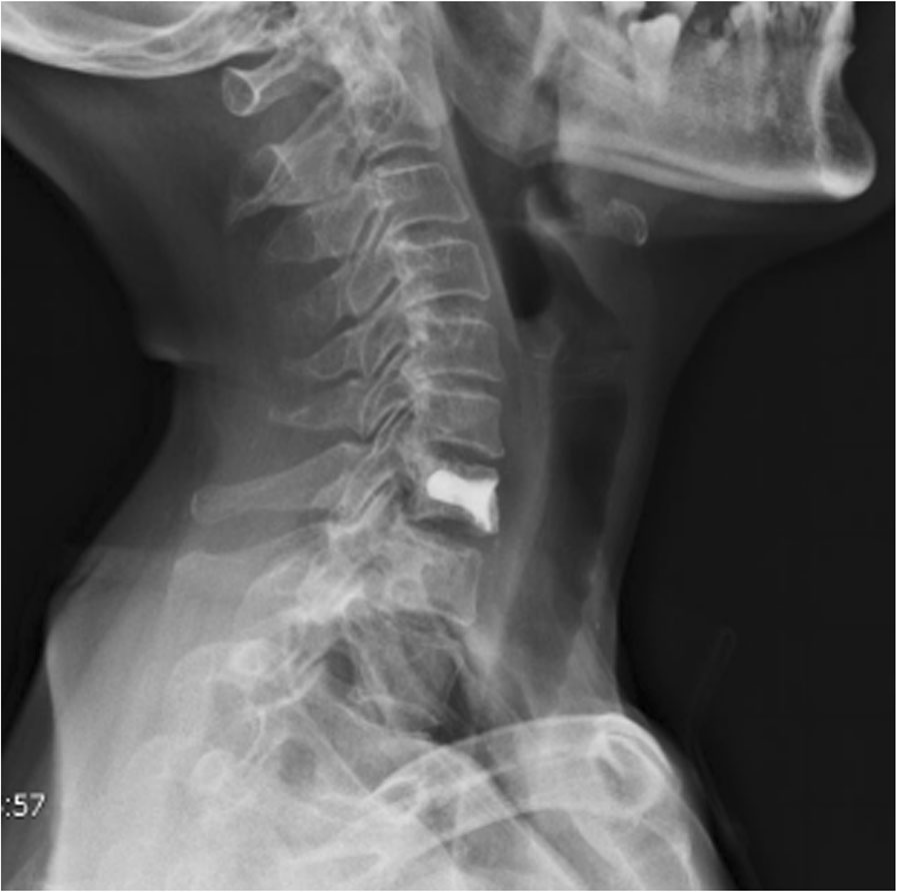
Six months after surgery imaging of X-ray
